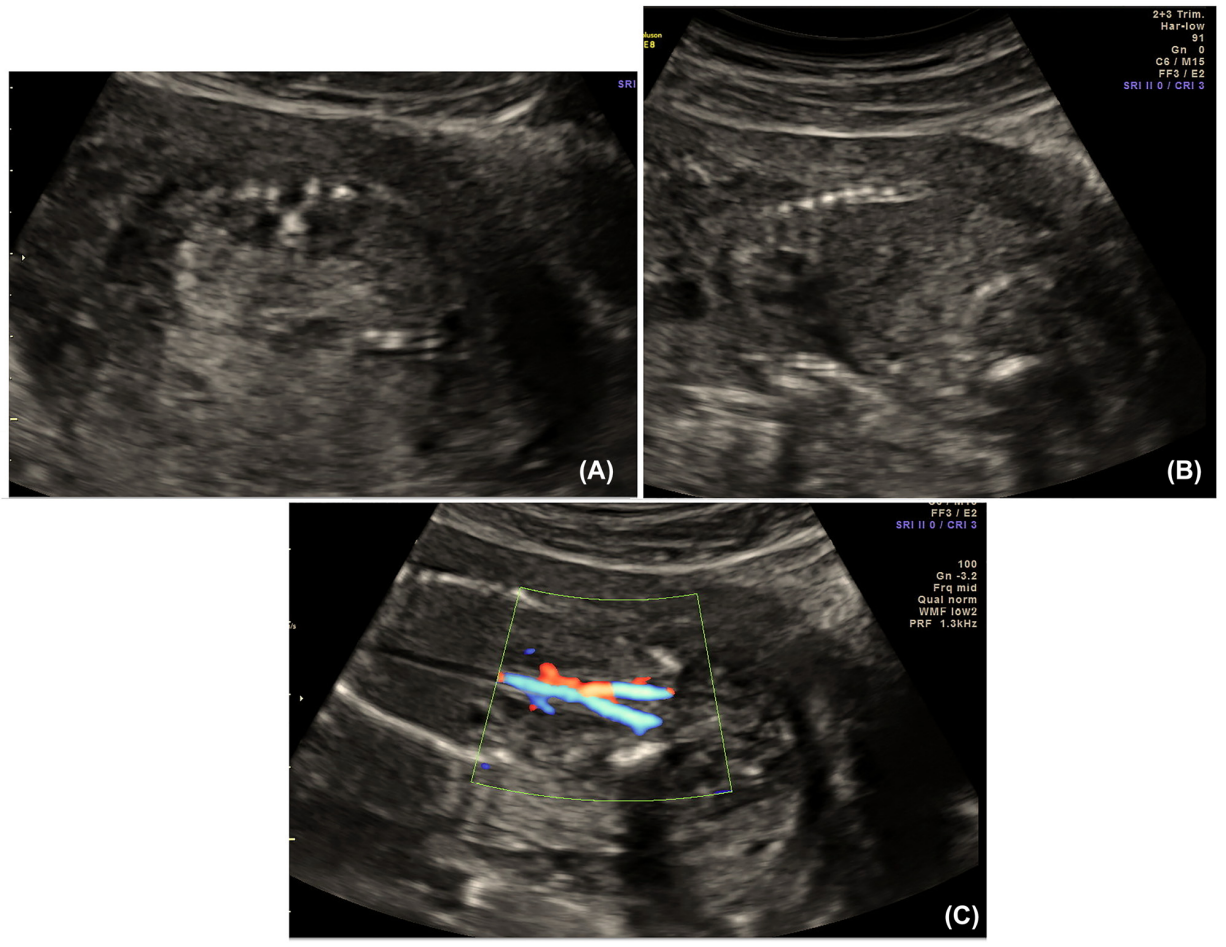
Ultrasound images of proband of family 1. (A) Anhydramnios and absent renal fossae. (B) Anhydramnios. (C) Absence of renal artery blood flow from aorta.
